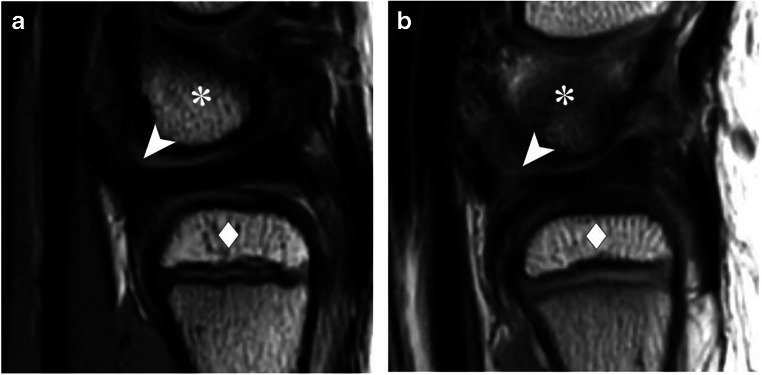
Sagittal proton-density weighted MRI showing the volar RUL as a structure that is a continuous and indiscernible with the TFC (arrowhead) and b discontinuous with the TFC (arrowhead) in between the ulnar head (diamonds) and lunate bone (asterisks)
